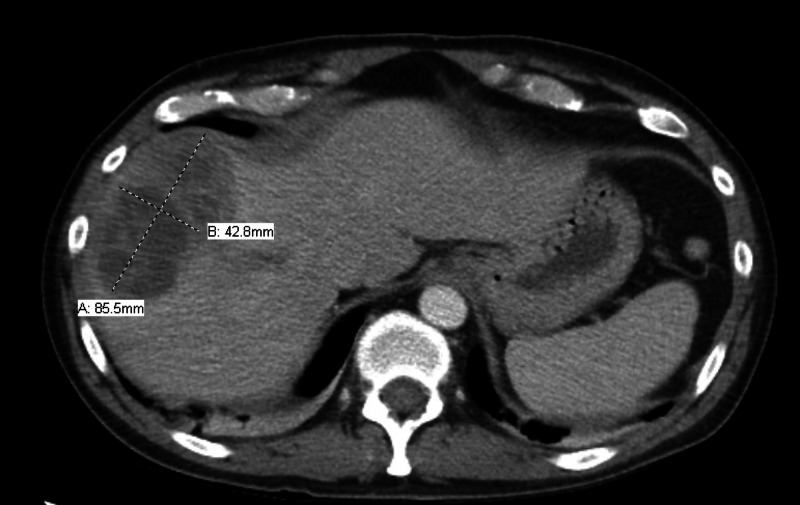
Initial CT scan of abdomen The cross mark on the left upper side shows hepatic abscess CT: computed tomography
